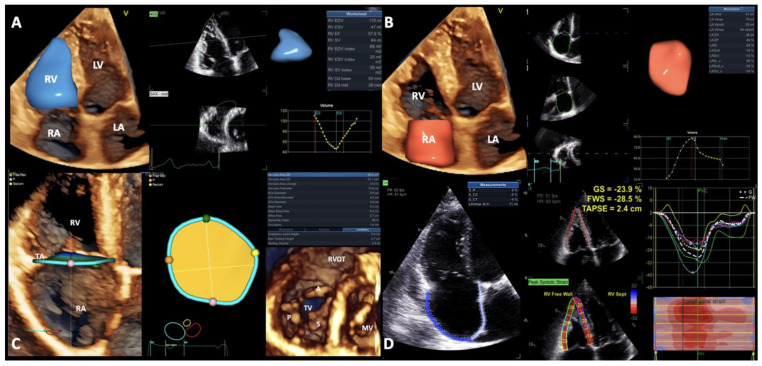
Right heart chamber and TA evaluation by 3DE and 2DSTE using dedicated software packages (EchoPac v204, GE, Horten, Norway). (A) RV volumes and function assessment using 4D Auto RVQ; (B) RA volume and function assessment by 4D Auto LAQ; (C) Tricuspid valve complex evaluation using 4D Auto TVQ; and (D) RA and RV strain analysis by AFI LA and AFI RV. Abbreviations: 2Ch—two chamber, 2D—two-dimensional, 2DSTE—two-dimensional speckle-tracking echocardiography, 3DE—three-dimensional speckle-tracking echocardiography, 4Ch—4 chamber, A—anterior leaflet, ED—end-diastole, ES—end-systole, FWS—free-wall strain, GS—global strain, LA—left atrium, LV—left ventricle, MV—mitral valve, P—posterior leaflet, PreA—before atrial contraction, PVC—pulmonary valve closure, RA—right atrium, RV—right ventricle, RVEDV—right ventricular end-diastolic volume, RV EF—right ventricular ejection fraction, RVESV—right ventricular end-systolic volume, RVOT—right ventricular outflow tract, S—septal leaflet, S_CD—conduit strain, S_CT—contractile strain, S_R—reservoir strain, TA—tricuspid annulus, and TV—tricuspid valve.
